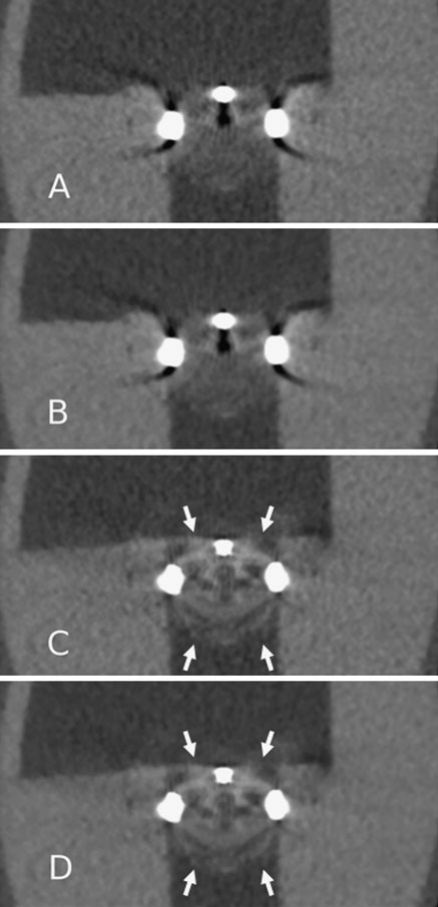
CT image reconstructions of Medtronic Hall tilting disc (Medtronic Inc., Minneapolis, MN, USA) with filtered back projection (a), iterative reconstruction (b), metal artifact reduction filter (c) and metal artifact reduction filter and iterative reconstruction (d). Note the secondary artifacts present in the MARF reconstructions (*arrows*) (c, d)
